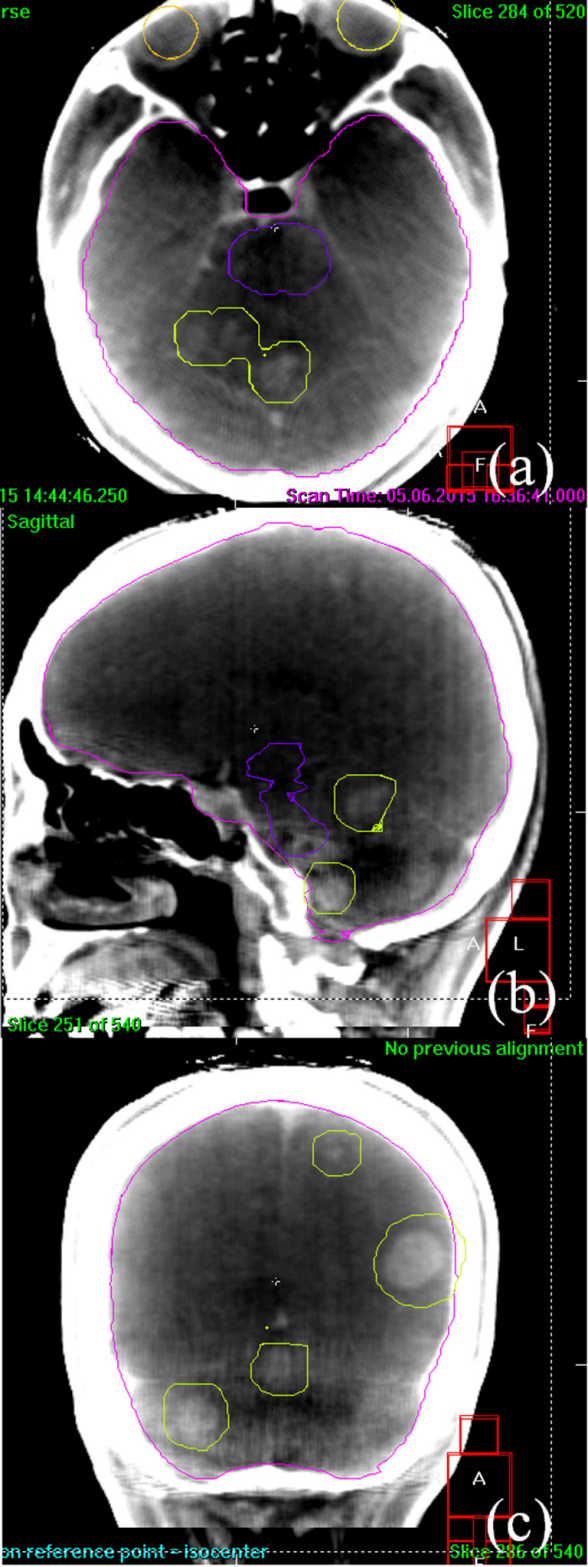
Contrast-enhanced in-treatment cone-beam CT images on (a) axial, (b) sagittal and (c) coronal planes during single-isocentre volumetric-modulated arc therapy acquired immediately after another intravenous bolus injection of iodized contrast media at the same dose as administered for the planning CT imaging. The planning target volume contours were also overlaid, thereby allowing us to directly verify the localization accuracy of each gross tumour volume.
